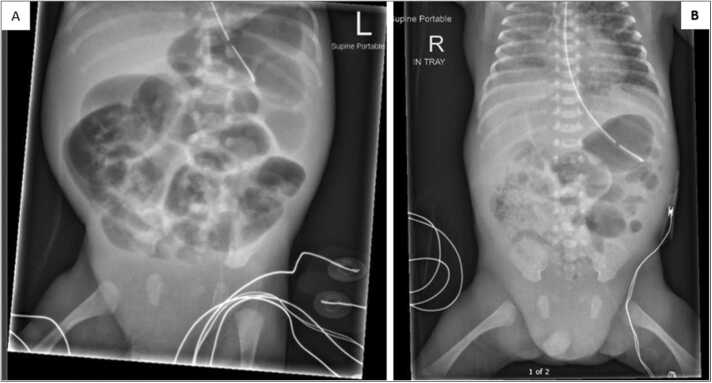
Simon’s abdominal x-ray demonstrating aerophagia (A) and perforation (B). (*Photo reproduced with family consent*).
